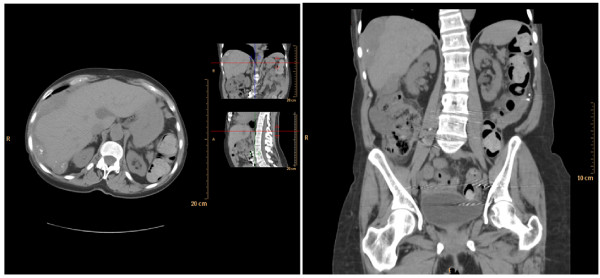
Cross-sectional computed tomography (CT) images displaying a right-sided, perihepatic soft tissue mass containing calcifications and subhepatic loculated fluid collection.
